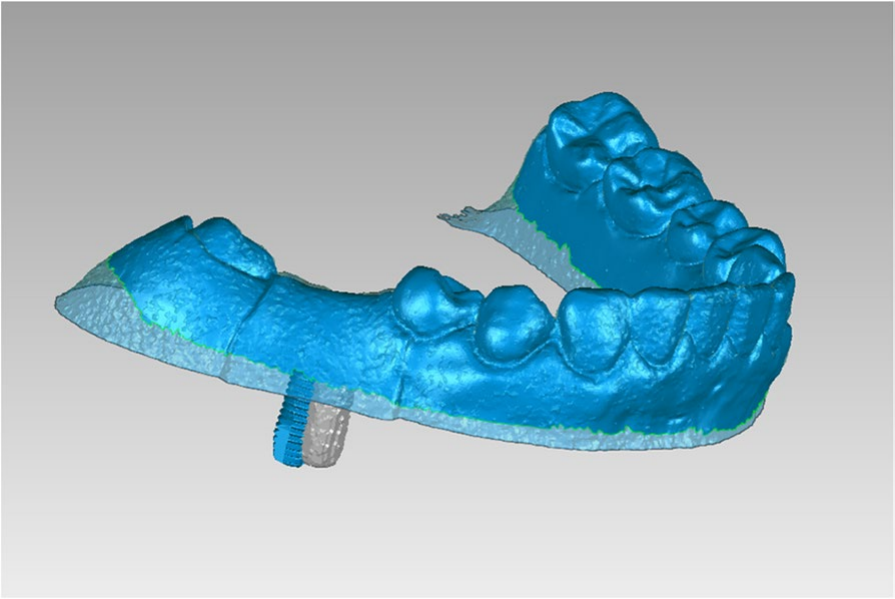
The STL files obtained from the presurgical plan and the postsurgical CBCT reconstruction were superimposed
